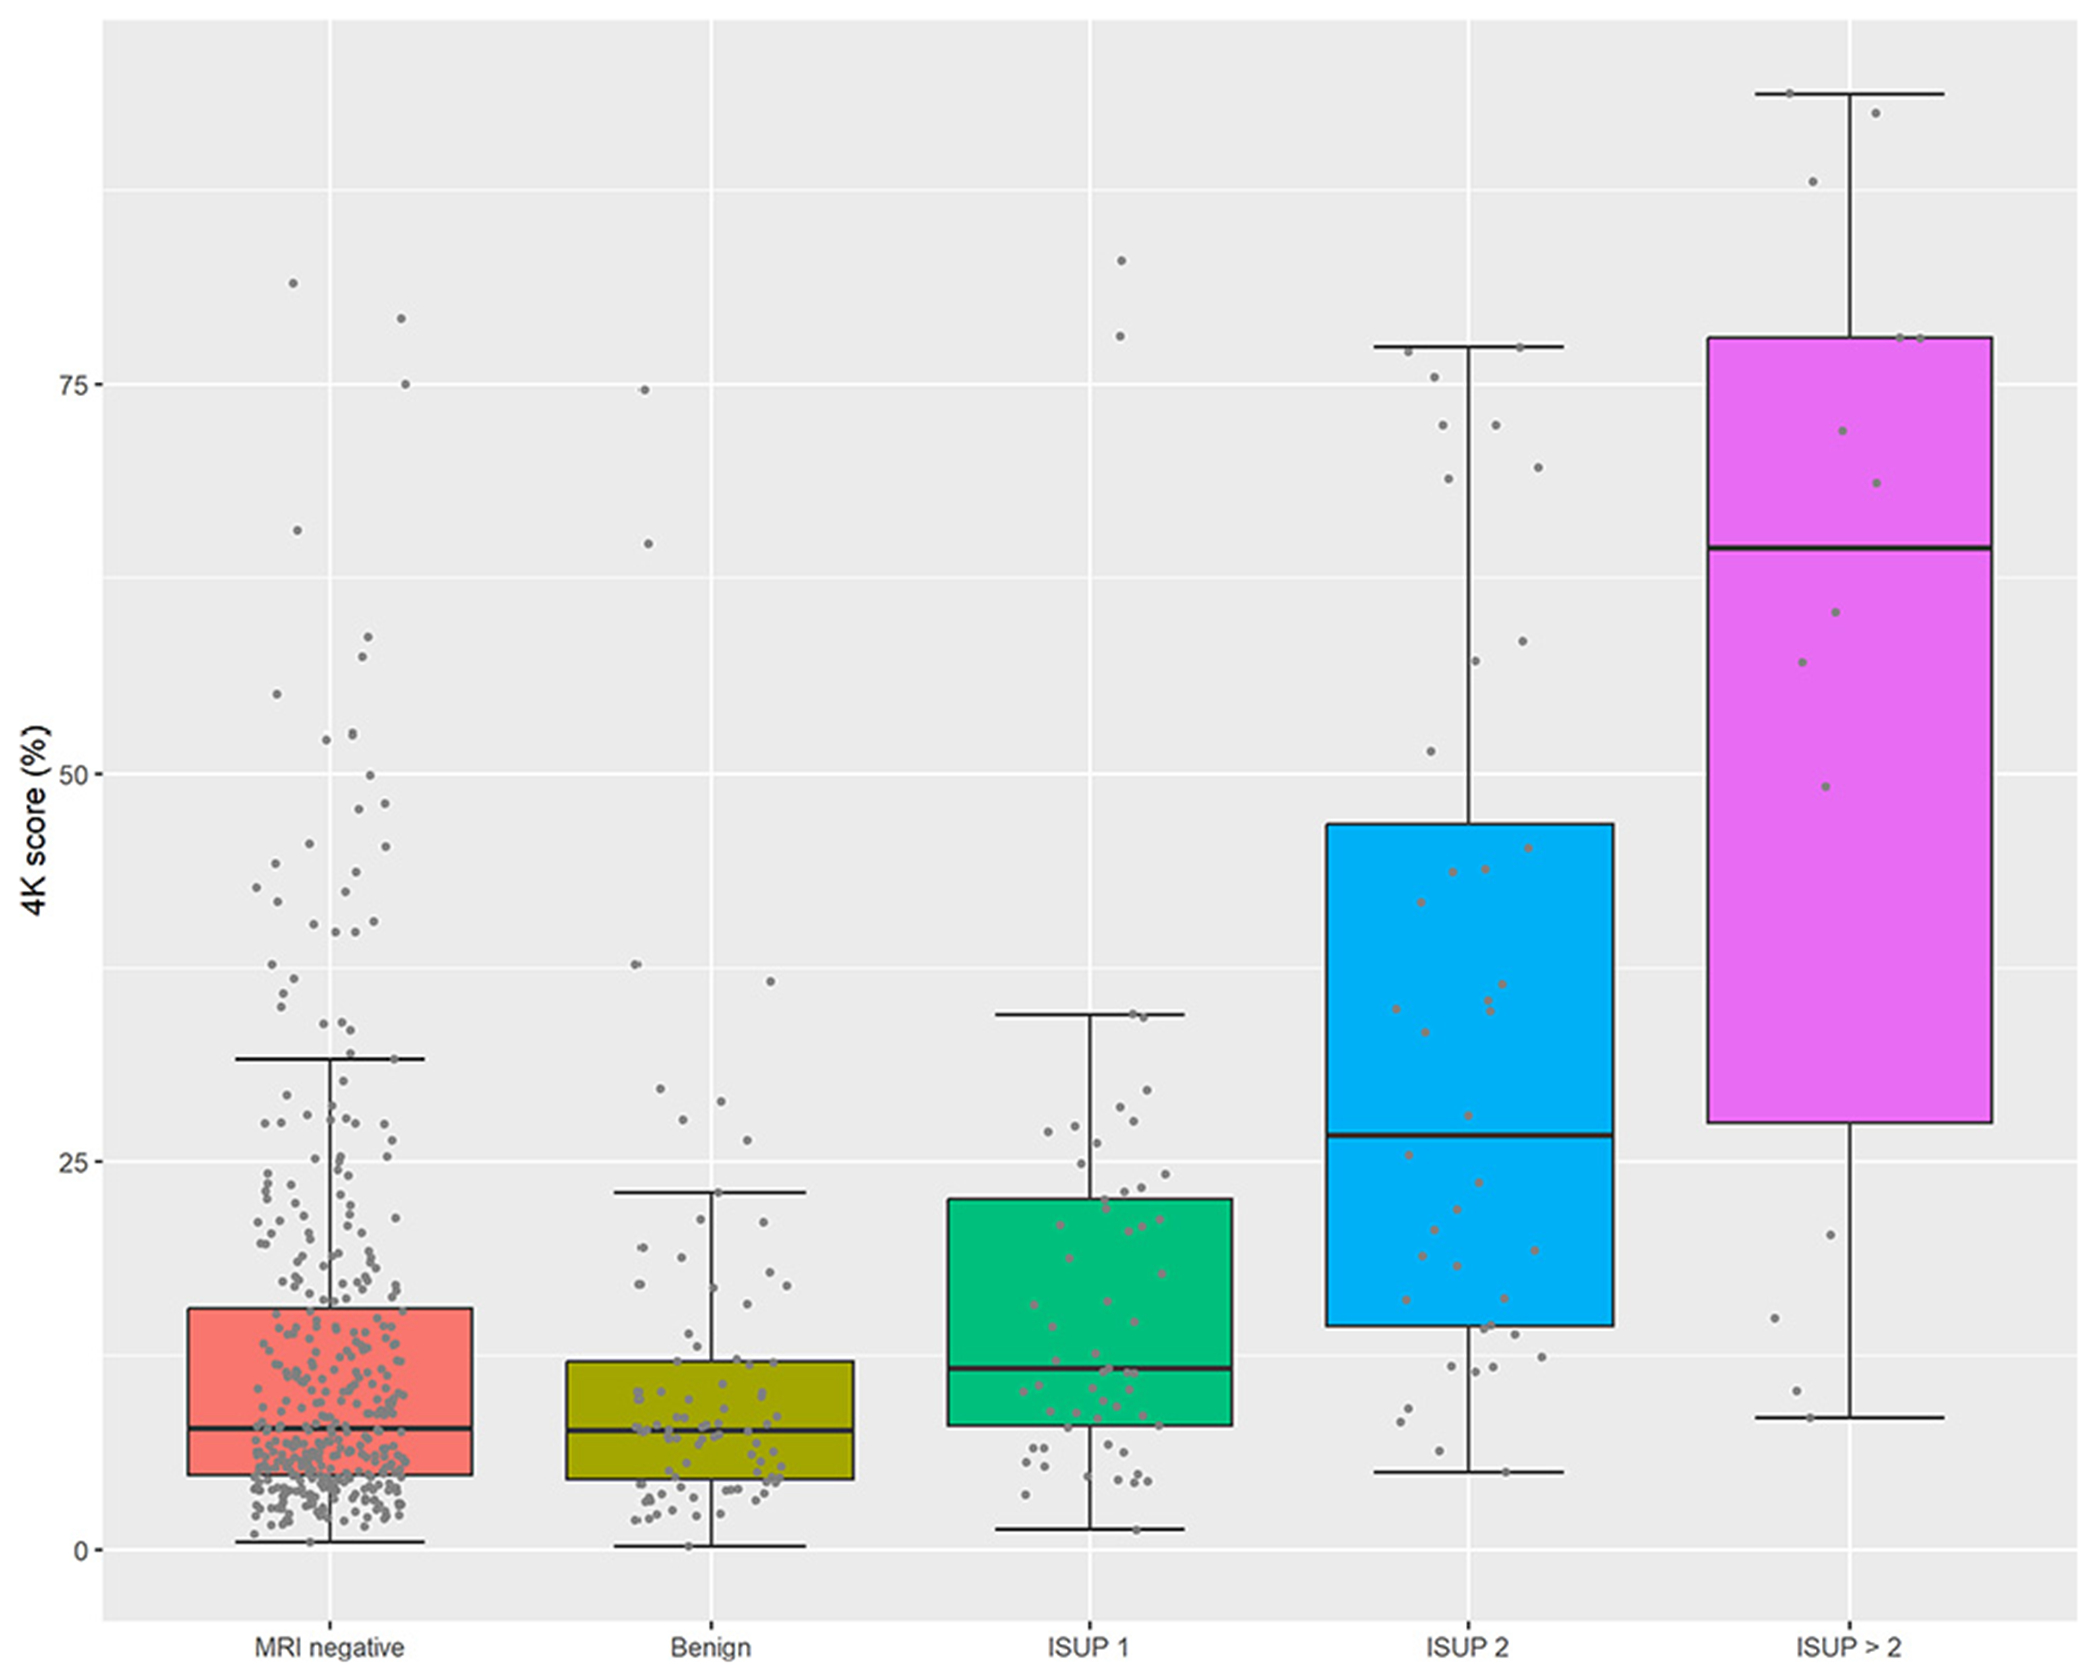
Box-dot plots of 4Kscore distribution by MRI and biopsy outcome. MRI = magnetic resonance imaging; ISUP = International Society of Urological Pathology; MRI = magnetic resonance imaging.
